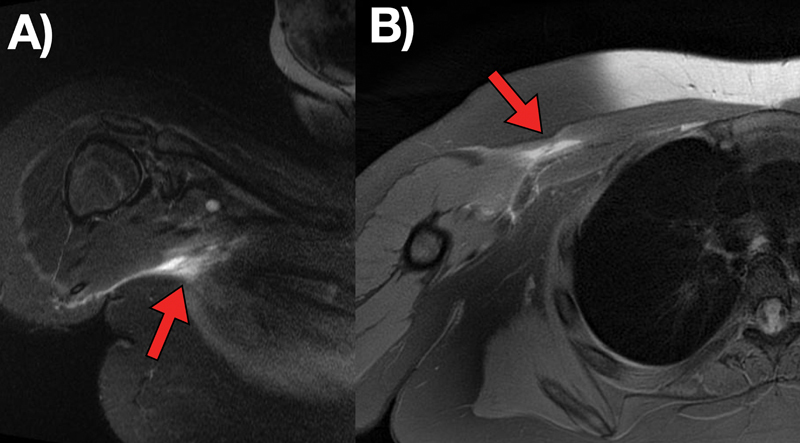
T2-weighted magnetic resonance imaging scans showing rupture of the pectoralis major muscle in the axial (
